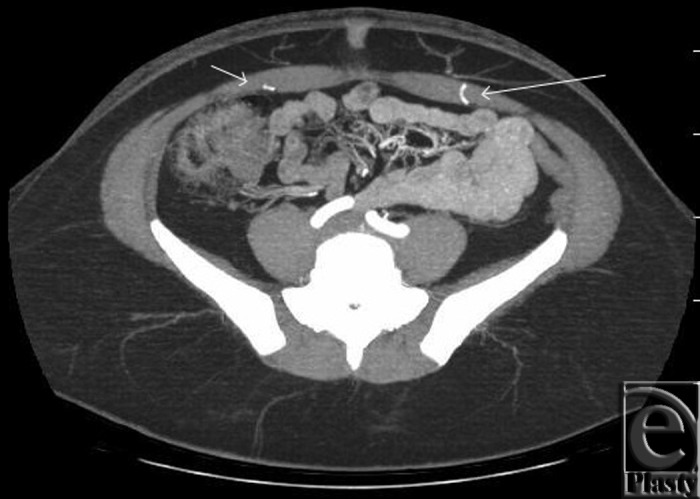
Right (short arrow) and left (long arrow) deep inferior epigastric perforators on preoperative computed tomographic angiography.
